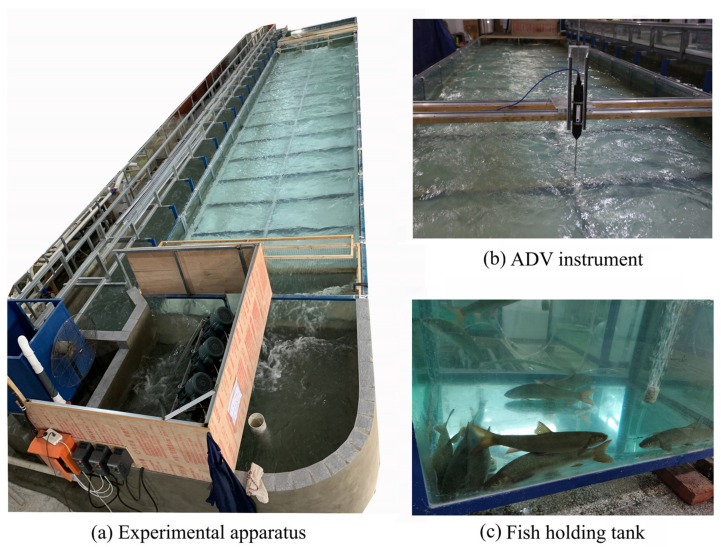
Real device: (a) experimental apparatus in the State Key Laboratory of Hydraulics and Mountain River Engineering of Sichuan University, (b) Acoustic Doppler Velocimeter (ADV) instrument, and (c) *Schizothorax prenanti* holding tank.
